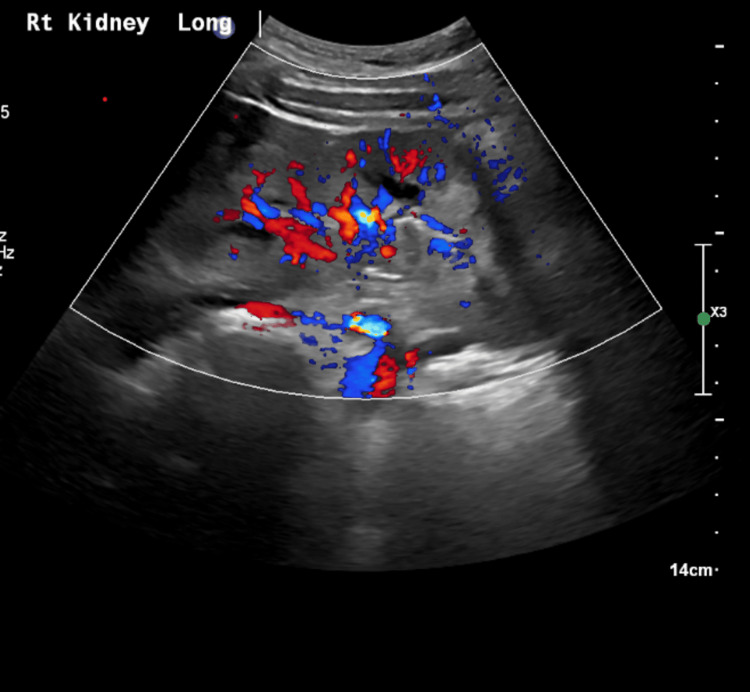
Doppler ultrasound of right kidney: Increased renal echogenicity, consistent with medical renal disease.
